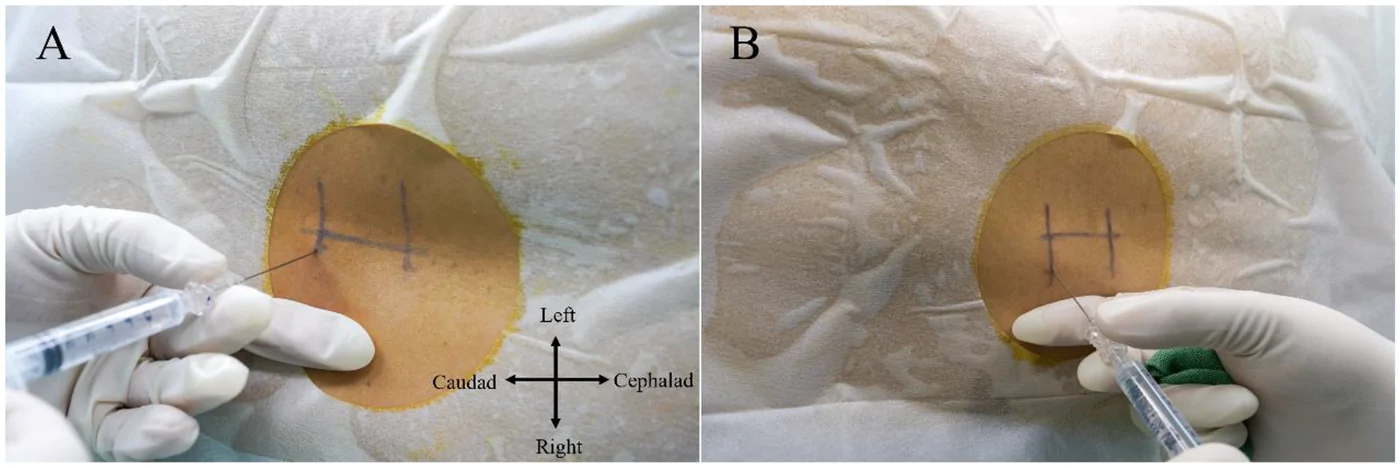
Comparison of needle insertion techniques between the modified and traditional paramedian approaches. (A) Ultrasound-assisted modified paramedian approach. The puncture point was located 0.5 cm lateral to the ultrasound-defined spinal midline on the non-operative side, and the spinal needle was advanced with a near-perpendicular trajectory relative to the skin surface. (B) Ultrasound-assisted traditional paramedian approach. The puncture point was located 1.5 cm lateral to the ultrasound-defined spinal midline on the non-operative side, and the spinal needle was advanced using the conventional oblique paramedian trajectory. Red dotted lines indicate the intended needle trajectories according to the predefined techniques.
